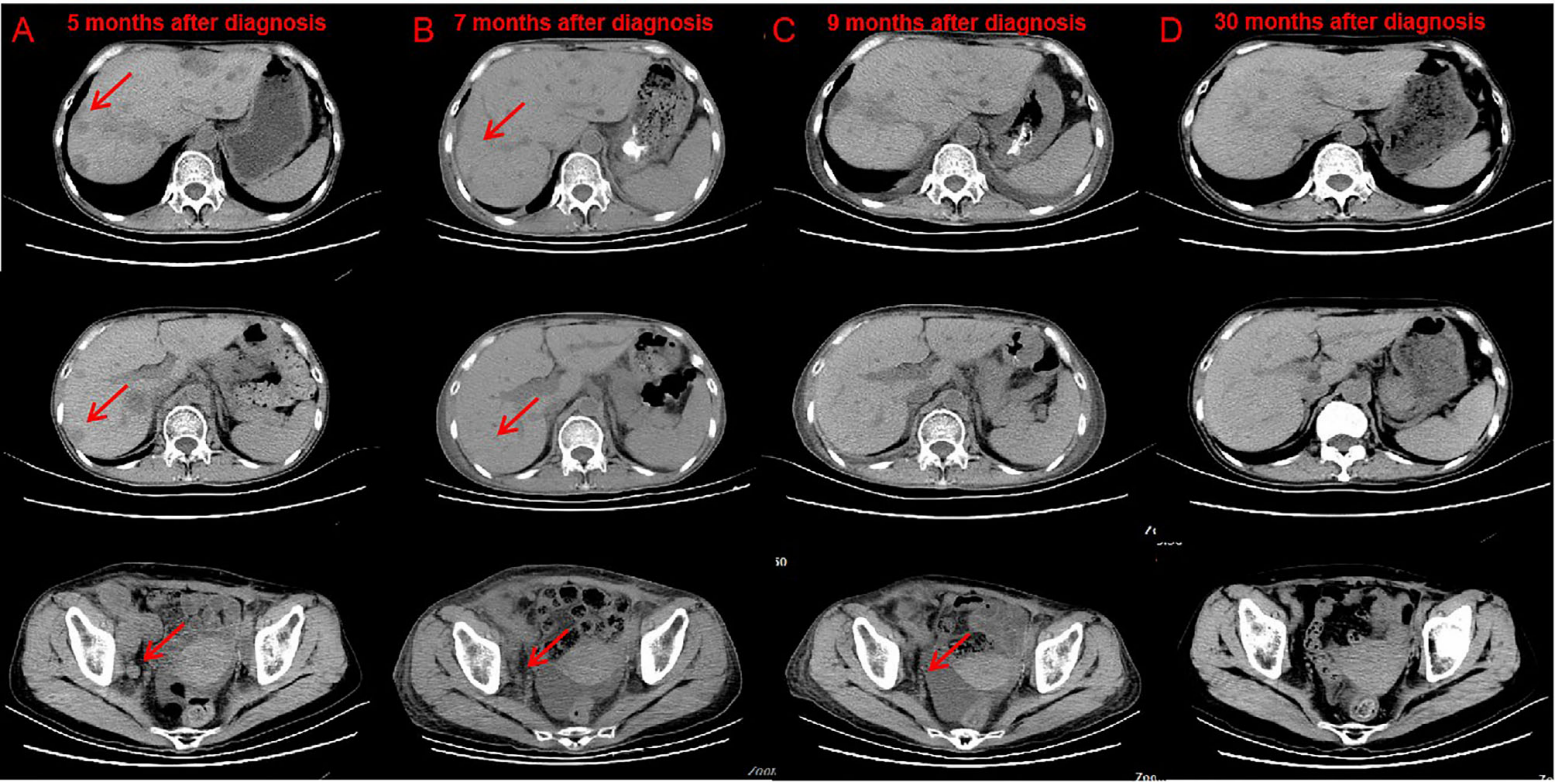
CT after nivolumab indicated tumor response. Compare CT of April 18, 2019 (B) with February 20, 2019 (A) Bladder cancer with liver, abdominal cavity, retroperitoneal, pelvic, bilateral inguinal lymph node metastasis, part of the liver lesions and lymph nodes shrink. CT of June 3, 2019 (C) and March 2, 2021 (D) showed no lesions were found in the liver, abdominal cavity, retroperitoneal, pelvic.
